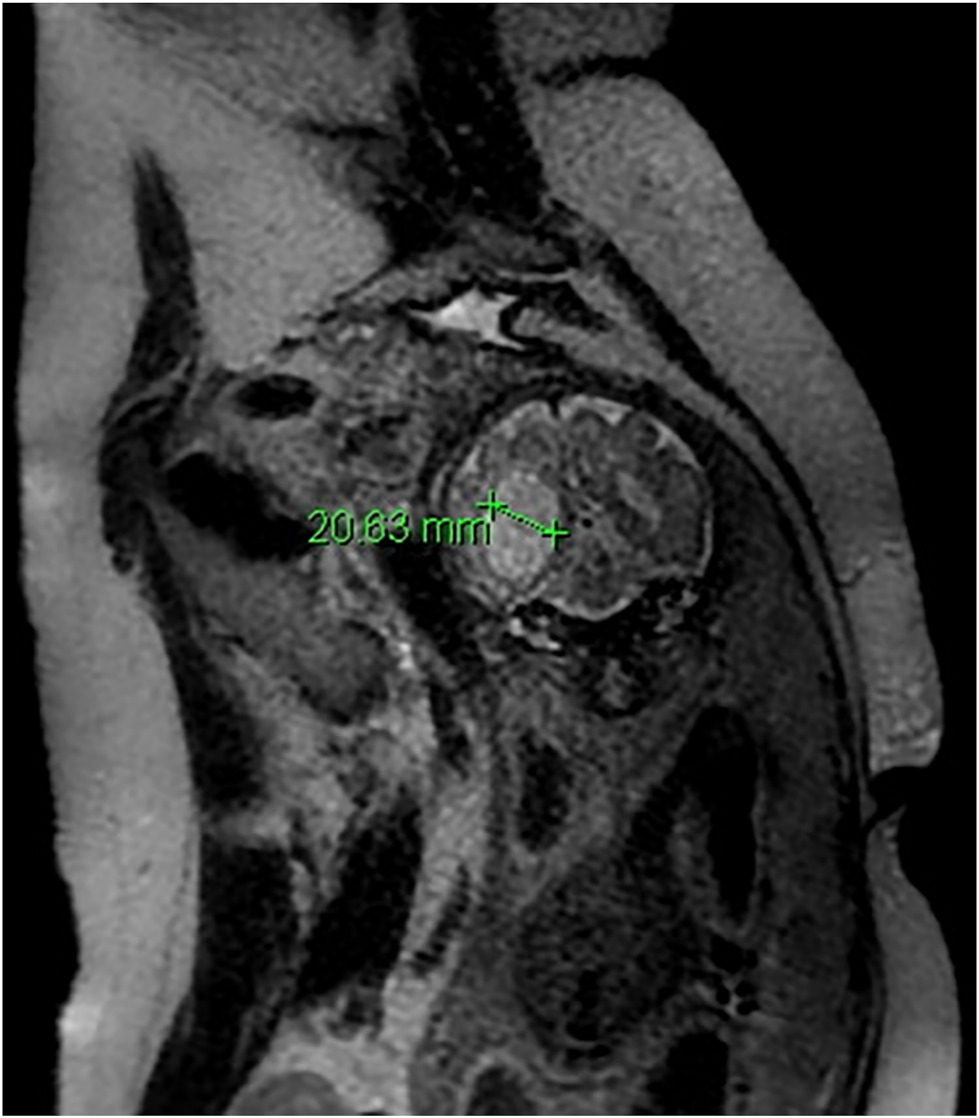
Fetal MRI at week 32 showing severe ventriculomegaly.
